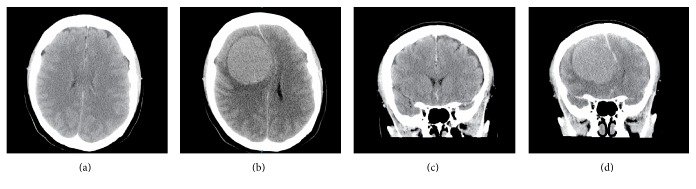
CT scan of the patient, displaying the rapid rate of growth of the meningioma: (a) axial view December 2012; (b) axial view December 2014; (c) coronal view 2012; (d) coronal view 2014.
